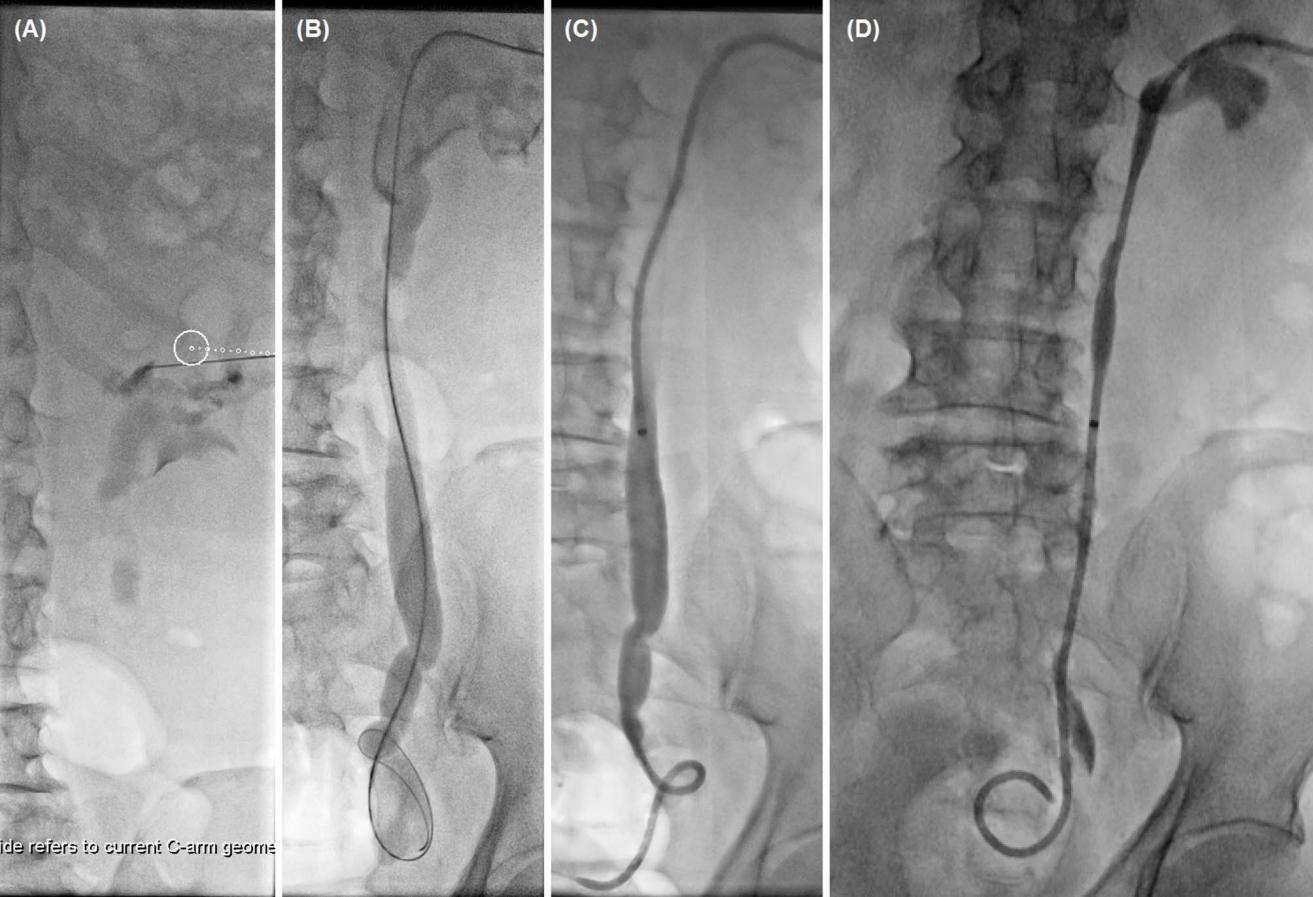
Percutaneous nephrostomy for 81-year male patient with right ureteral carcinoma. (A) Percutaneous renal pelvis puncture was performed under the virtual navigation of c-arm CT iGuide and fluoroscopic guidance. (B) A 0.035 inch guidewire and a 5 F catheter were passed through the ureteral occlusion segment. (C) A 8.5 F drainage tube was inserted for urine drainage. (D) Urothrography was performed again after 1.0 month
